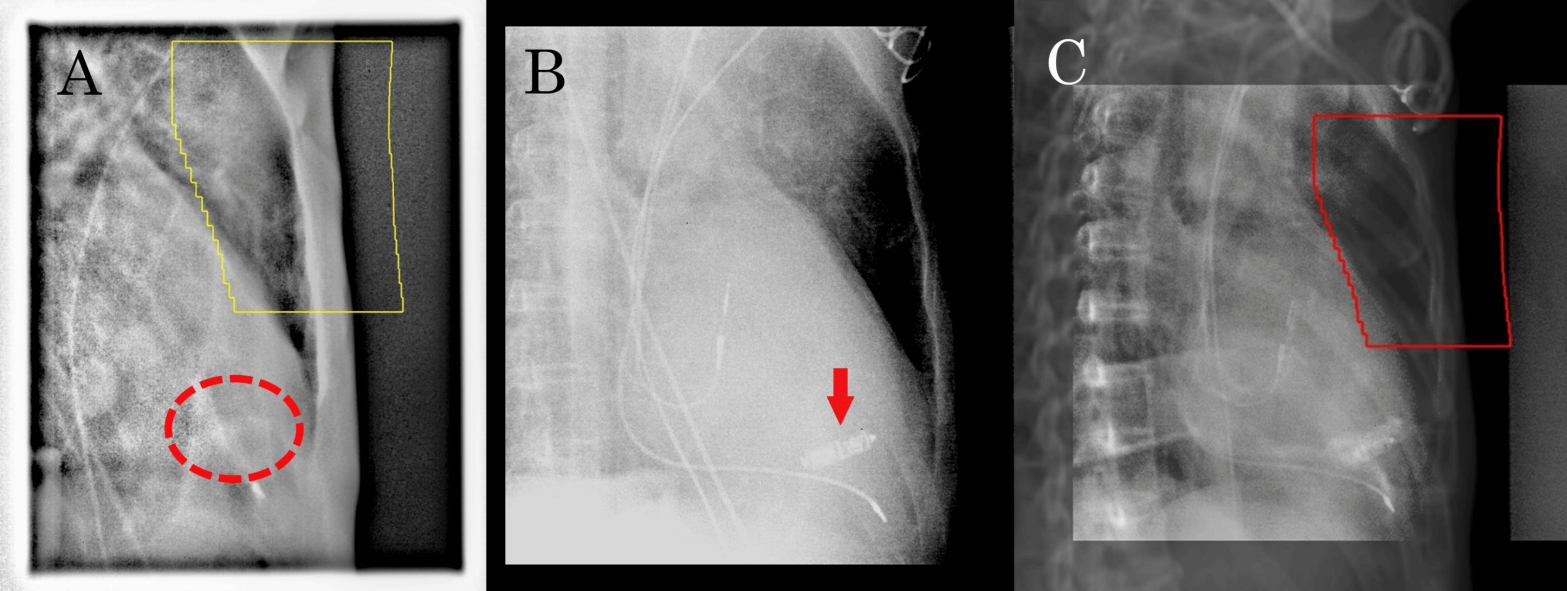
Image-guided verification of pacemaker position (A) Electronic portal imaging on the day before treatment. The expected location of the leadless pacemaker (LPM), outlined by a red dashed circle, is not clearly visible. The yellow contour indicates the radiation field. (B) Kilovoltage setup image on the same day. Residual pacing leads from the previous device are seen. The red arrow indicates the LPM. Using fluoroscopic mode, the LPM was confirmed to move outside the treatment field. (C) Image registration of the digitally reconstructed radiograph and kilovoltage image on the treatment day. The red contour indicates the radiation field.
